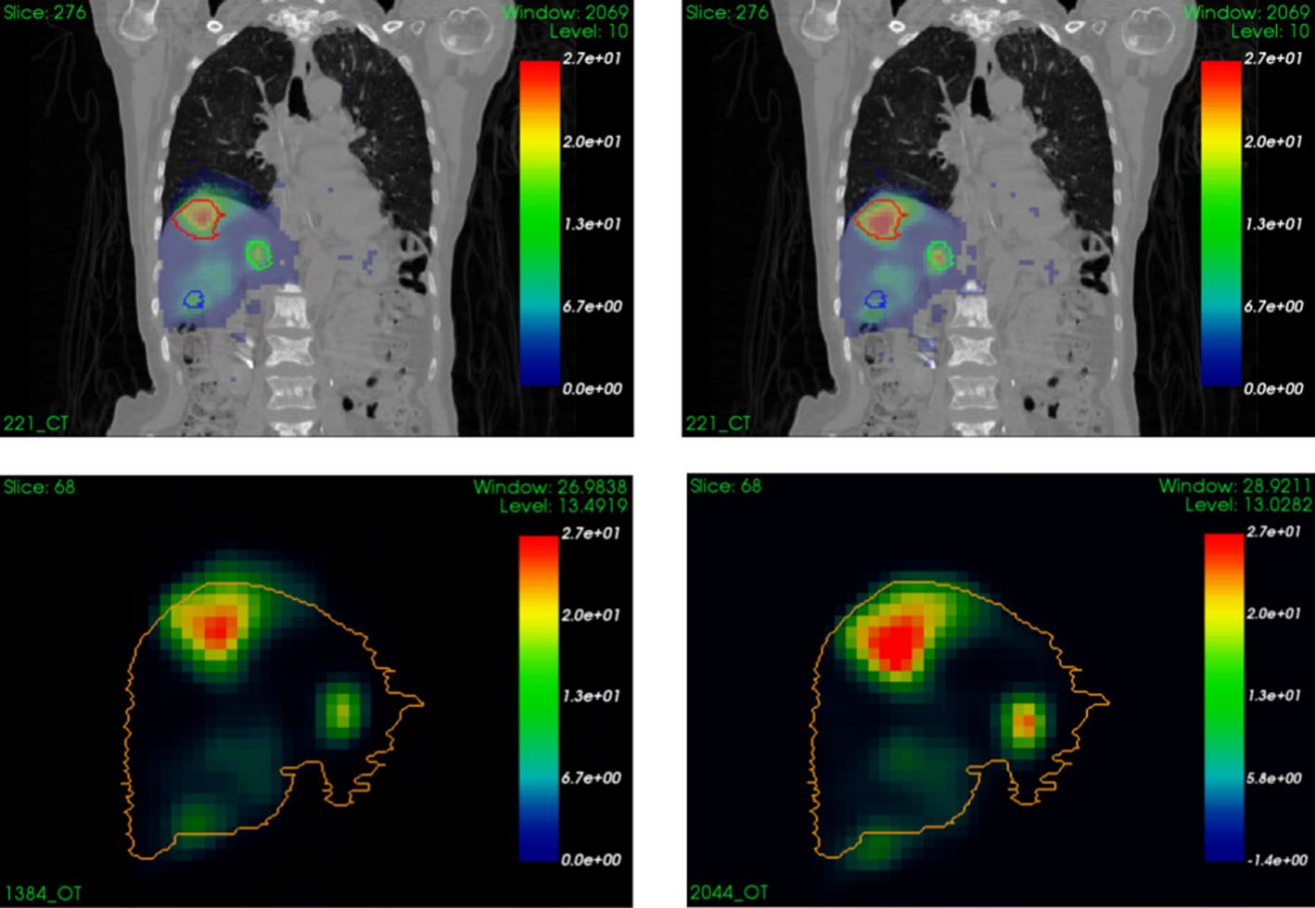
3D (left) and 3Dcomp (right) reconstructions overlapped on CT (top) and reconstructions alone. Red, green and blue contours correspond to lesion contours initially drawn on the CT image. Orange contour is the mask of the liver
